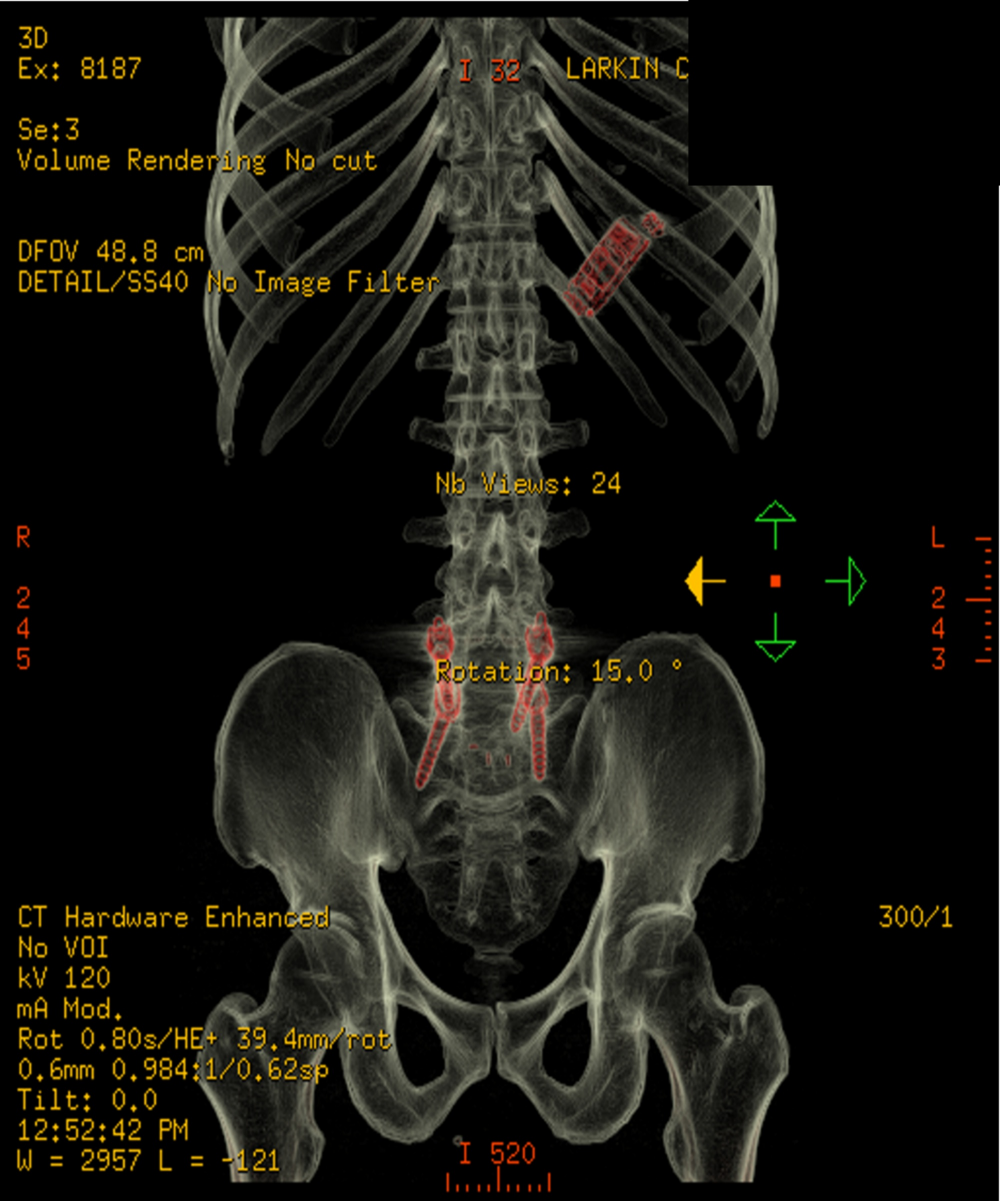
Hardware-enhanced CT. The foreign body is much more clearly delineated here using hardware-enhanced CT.
